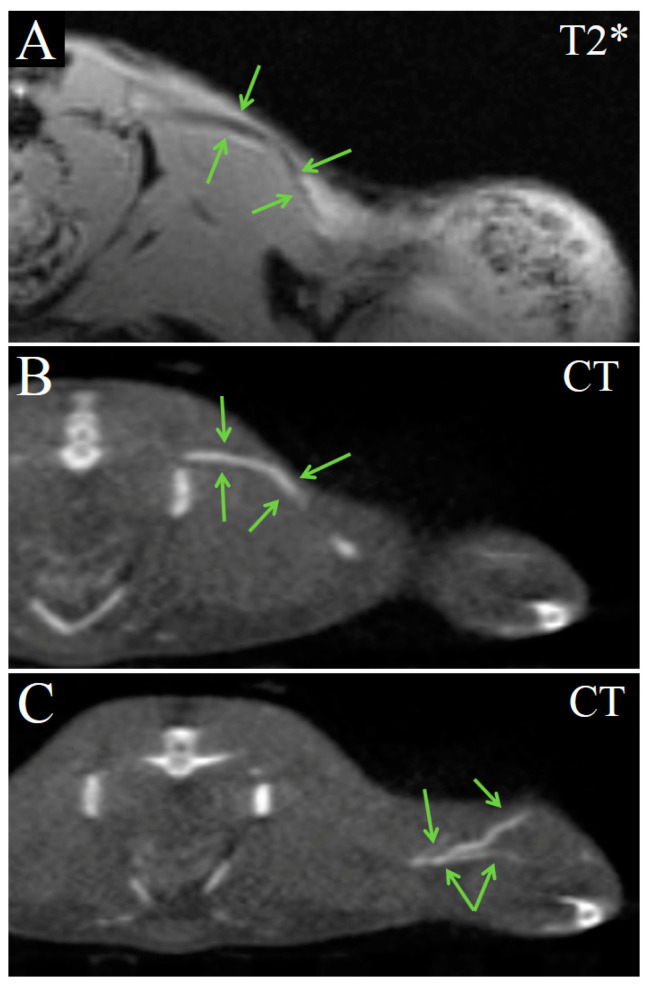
Transversal images demonstrate the enhancement of blood vessels. (A) T2* MRI 16 min post injection of Fe_3_O_4_@Au; (B) CT image 22 min post injection of Fe_3_O_4_@Au; (C) CT image of the tumor 22 min post injection of Fe_3_O_4_@Au. Arrows indicate blood vessels.
